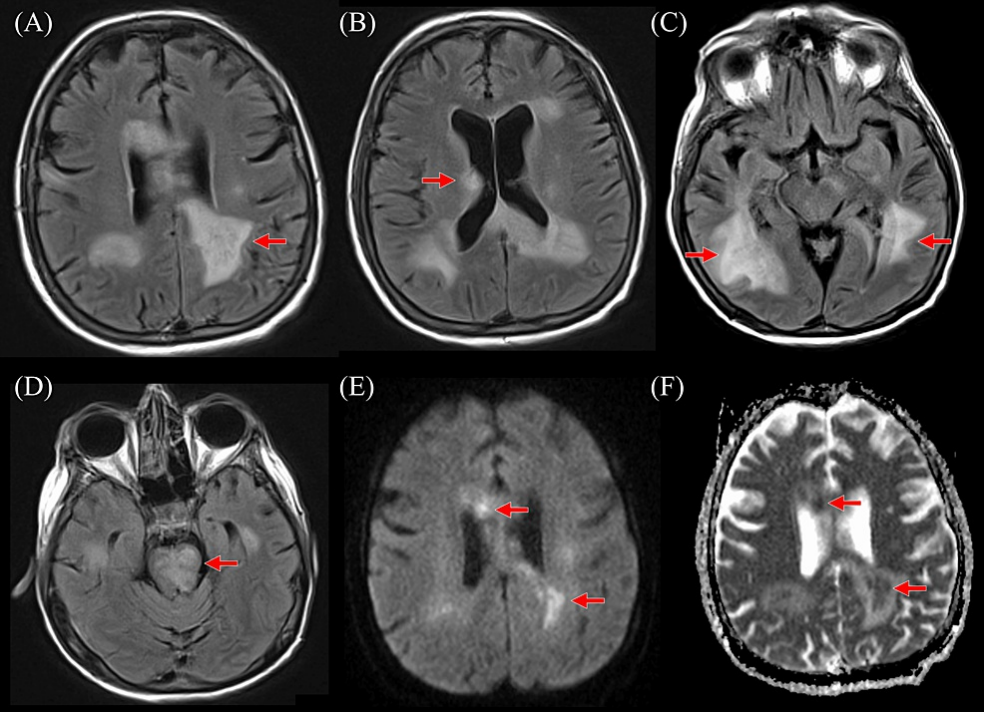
Axial FLAIR images on the first follow-up MRI at the (A) parietal white matter, (B) ganglionic, and (C) temporal lobes, and (D) pons levels demonstrate increased confluent FLAIR hyperintensities and central areas of restricted (E) diffusion with (F) ADC correlate *Abbreviations:* ADC = Apparent Diffusion Coefficient, FLAIR = Fluid-Attenuated Inversion Recovery
